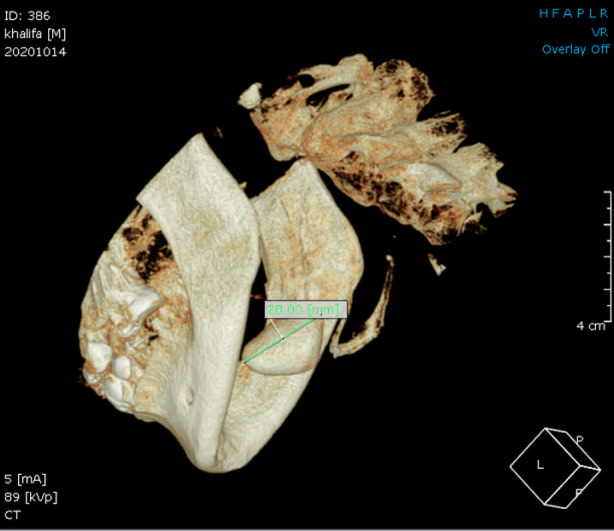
The 3D reconstructed cone beam computed tomographic (CBCT) image shows a well-defined calcified mass in the right submandibular glandular region with the maximum dimension being 28mm.
